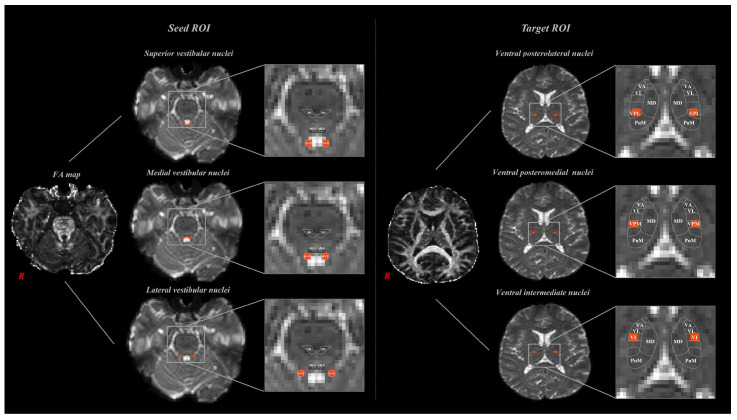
Seed region of interest (ROI) and Target ROIs set in magnetic resonance imaging (MRI) analysis. Seed ROIs were placed in the vestibular nuclei (SuVN, MVN, LVN) in the brainstem, and target ROIs were placed in the thalamic nuclei (VPL, VPM, VI).
